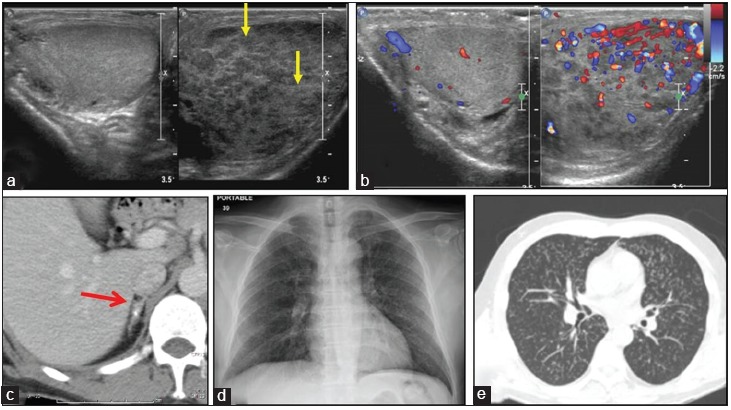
Testicular tuberculosis mimicking tumor; importance of extratesticular ancillary findings. (a) Gray scale sonographic image of scrotum showing enlarged left testis with multifocal ill-defined hypoechoic nodularity (yellow arrows). (b) Color Doppler shows increased vascularity. The left radical orchiectomy revealed caseous granulomatous orchitis and epididymitis. (c) Retrospective analysis of contrast-enhanced computed tomography (CT) abdomen shows calcified right adrenal gland (red arrow). (d) Chest X-ray was normal and (e) CT chest revealed bilateral lung parenchyma studded with multiple miliary tubercles.
